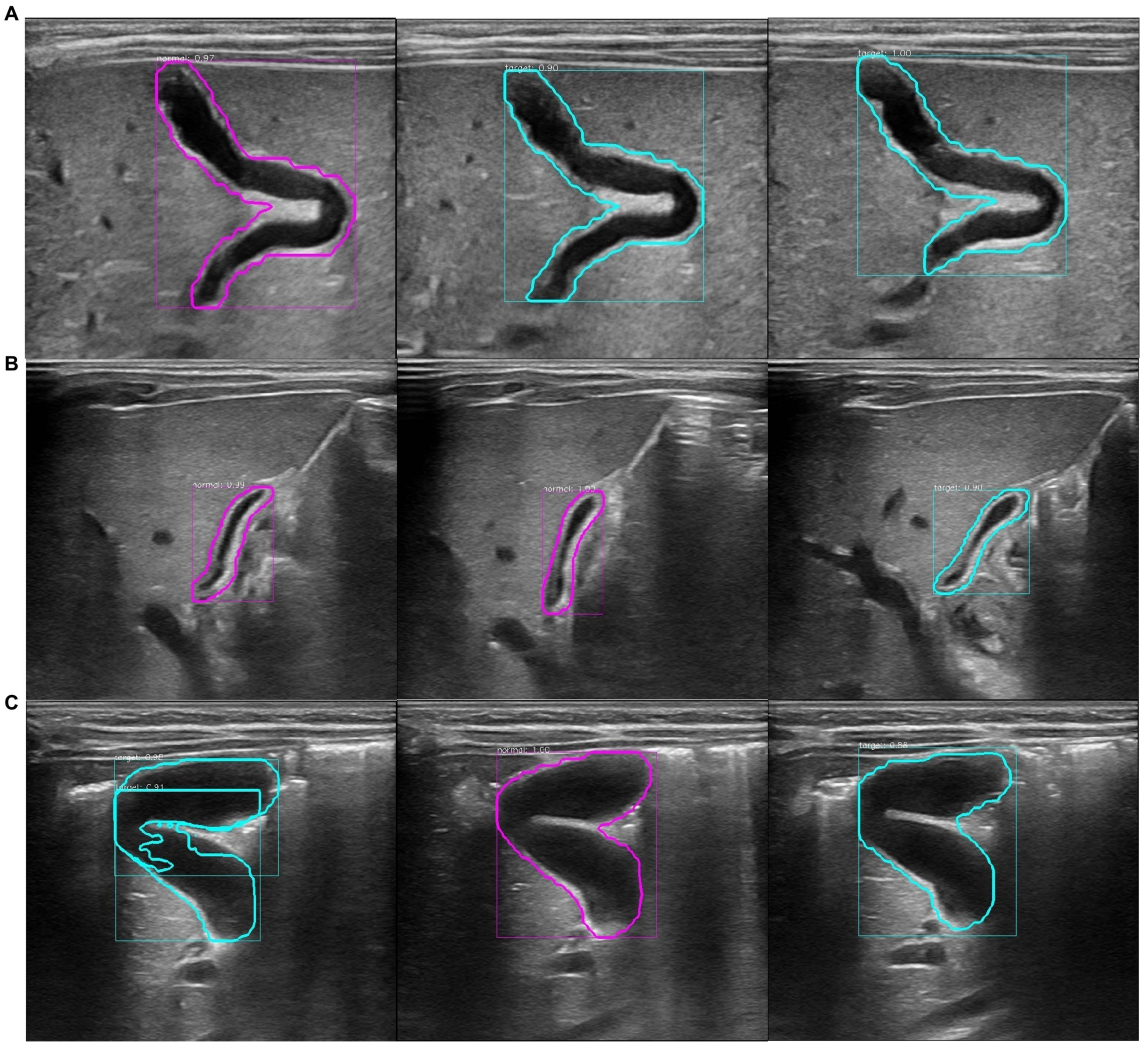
Recognition results of the model at the patient level. The purple circle in the figure represents the recognition result of a single image as non-biliary atresia. The blue–green circle represents the recognition result of a single image as biliary atresia. (A) Ultrasound images of the gallbladder of the same patient with biliary atresia. The recognition result shows that the average probability of biliary atresia is approximately 0.64, and the recognition result is correct. (B) Ultrasound images of the gallbladder of the same patient with non-biliary atresia and hyperbilirubinemia. The model recognition result shows that the average probability of non-biliary atresia is approximately 0.70, and the recognition result is correct. (C) Ultrasound images of the gallbladder of the same healthy infant. The model recognition result shows that the average probability of biliary atresia is approximately 0.62, and the recognition result is incorrect.
